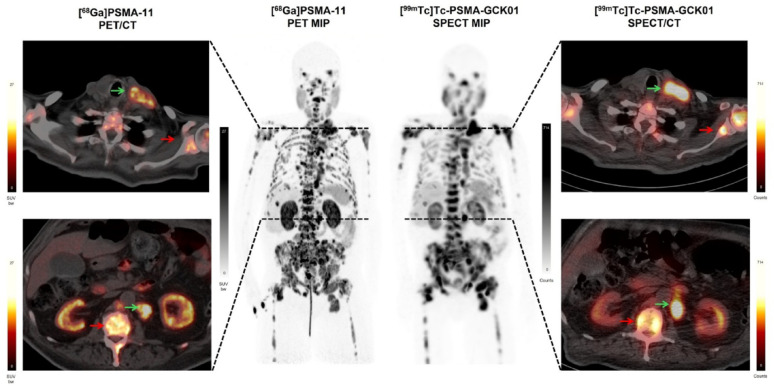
A 67-year-old patient with disseminated bone metastases in advanced stage of prostate carcinoma underwent both PSMA PET/CT and SPECT/CT scans for determination of phenotyping prior to RLT. Red arrows indicate bone metastases; green arrows indicate lymph node metastases. Interestingly, the high tracer uptake is also observed in the Virchow lymph nodes
